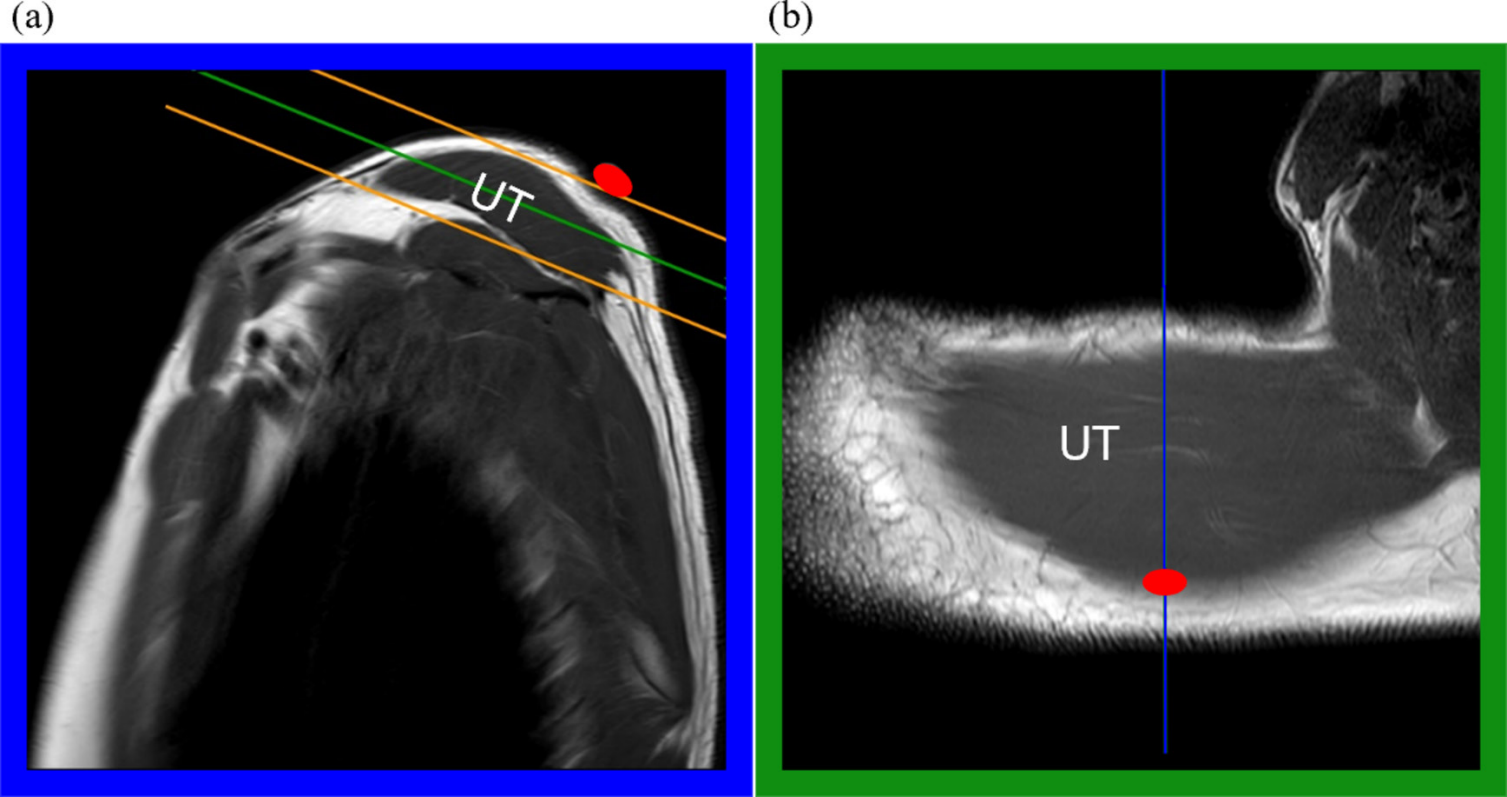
Localisation of UT in the MR images. The blue line in (b) corresponds to the position of the sagittal section in (a). A series of oblique axial images were prescribed to cover the entire UT muscle between the orange lines in (a). The green line in (a) corresponds to the position of the oblique axial section of UT in (b) which is obtained at a depth of approximately 1 cm beneath the subcutaneous tissue. The position of the border between UT and MT is marked by the fish oil capsule denoted by a red symbol.
